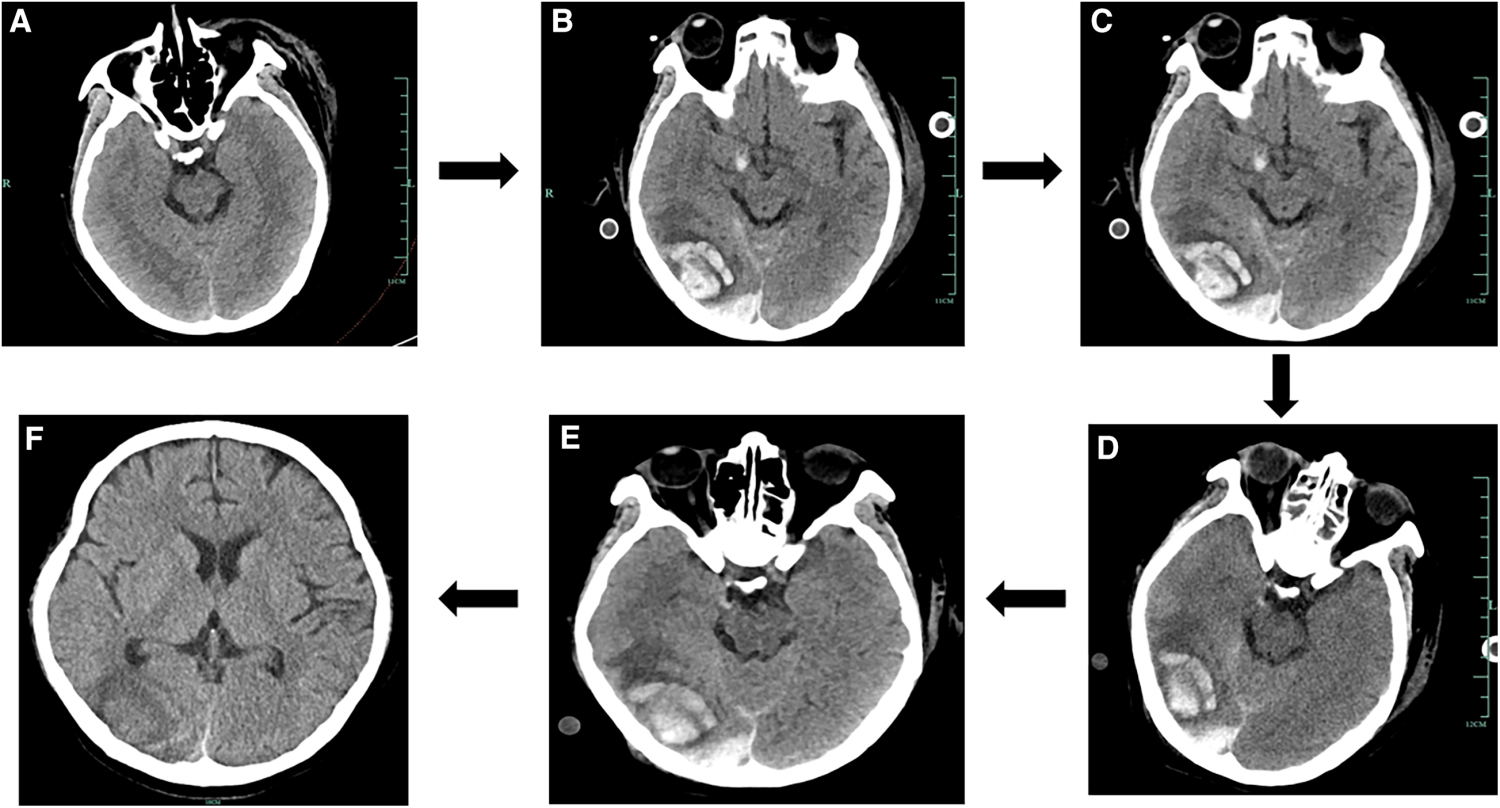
Results of the cranial CT scans during the patient's hospital stay. (A) Results of the patient's cranial CT on Day 1. (B) Results of the patient's cranial CT on Day 6. (C) Results of the patient's cranial CT on Day 9. (D) Results of the patient's cranial CT on Day 11. (E) Results of the patient's cranial CT on Day 15. (F) Results of the patient's cranial CT on Day 35.
